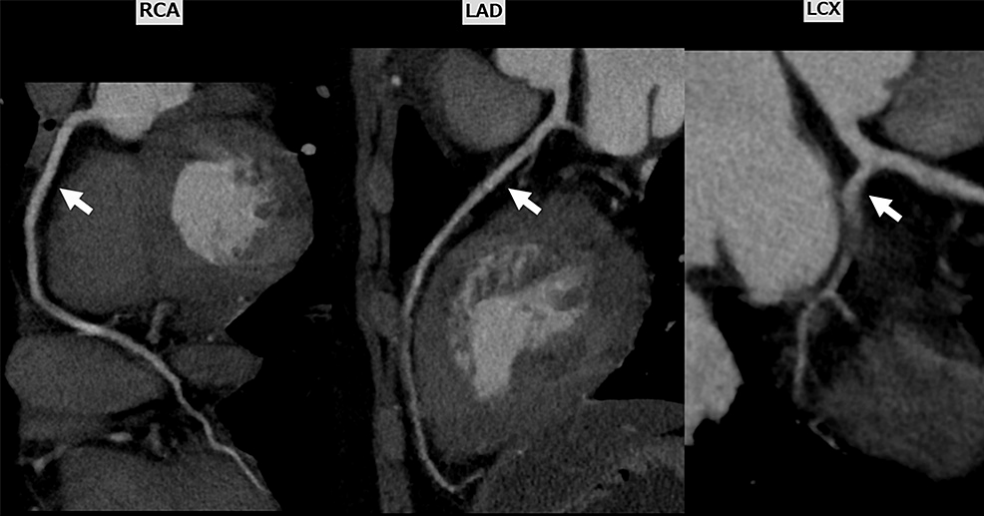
Coronary computed tomography angiography showing no stenosis of the coronary arteries. RCA, right coronary artery; LAD, left anterior descending artery; LCX, left circumflex artery.
